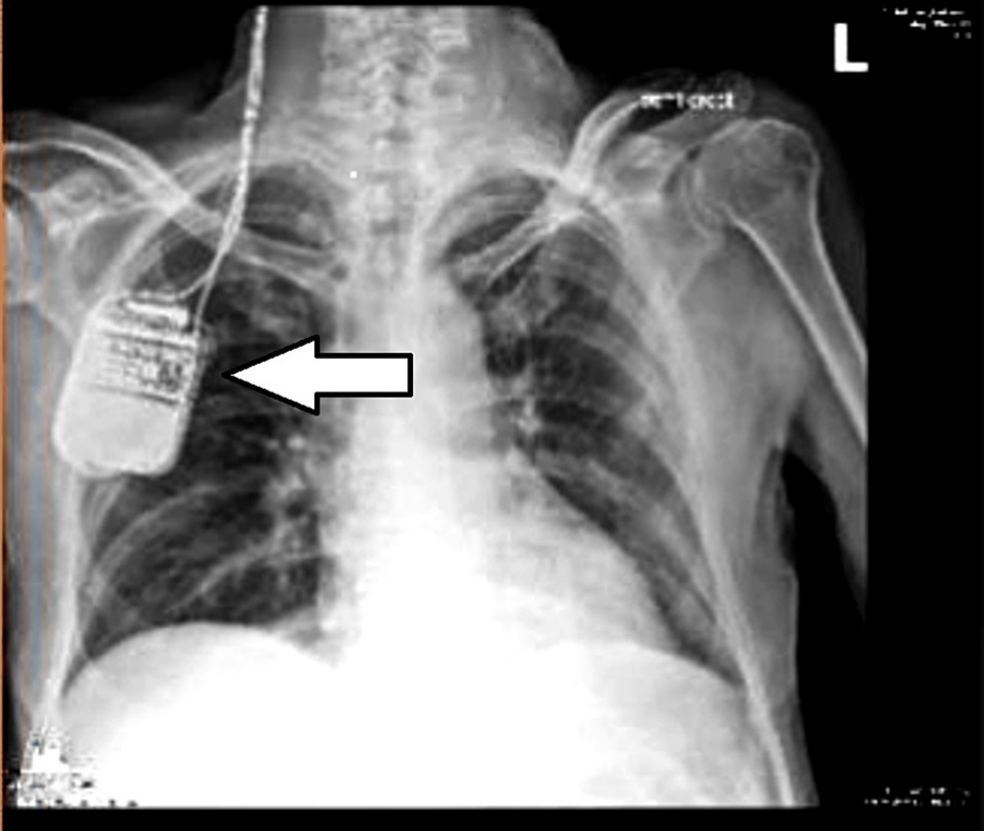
Chest radiography showing the internal pulse generator of the deep brain stimulator device
